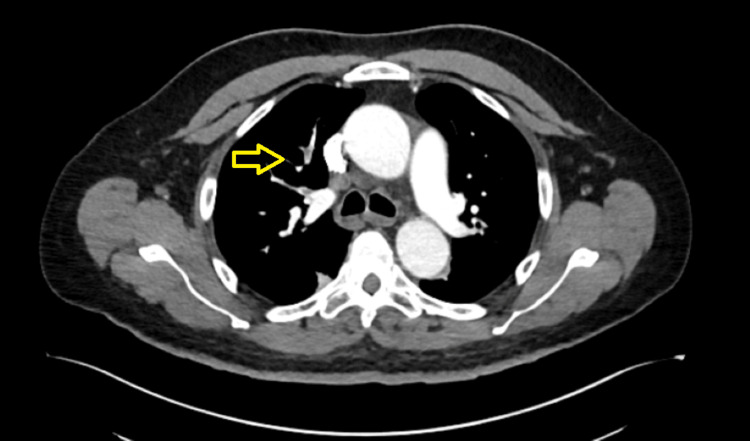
CT pulmonary angiography showing the right upper lobe pulmonary embolism.
